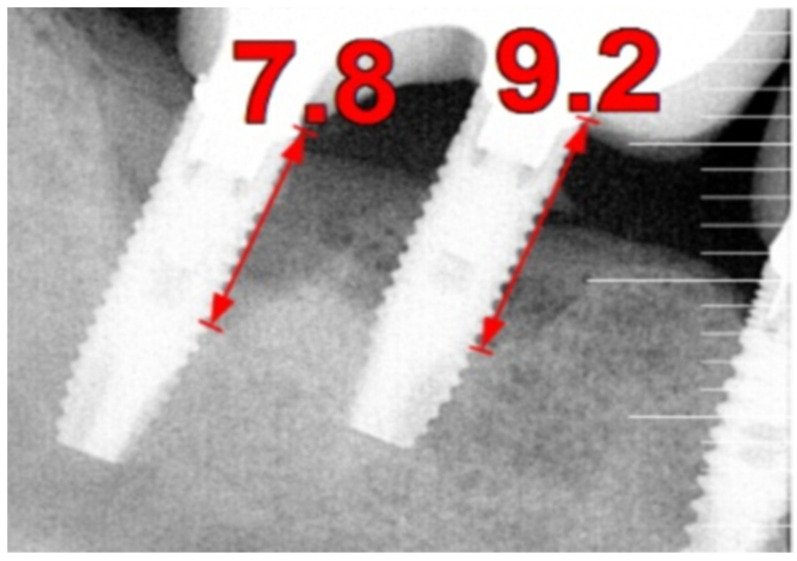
The periapical image taken for #46 and #47 (region of the complaint) shows relevant bone loss around the implants (red arrows showing the bone loss found, considering as reference the implant platform).
